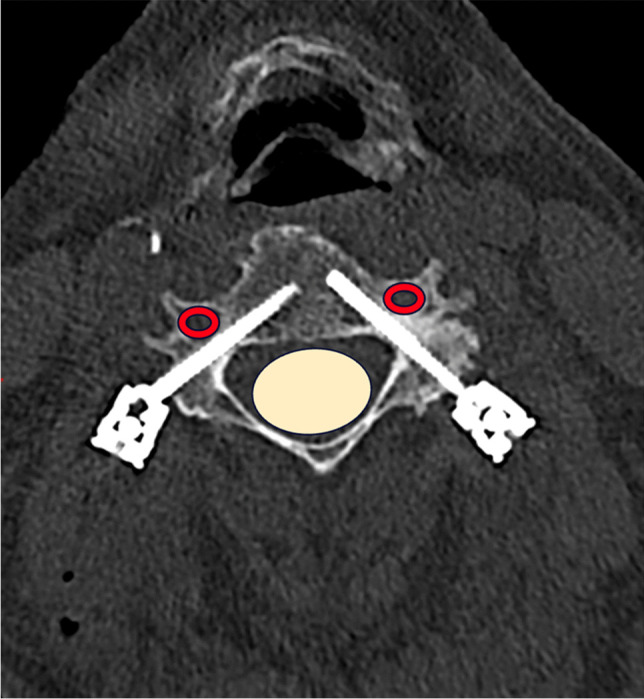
Postoperative CT image in the axial plane showing cervical pedicle screws in place, the vertebral arteries are marked in red, the spinal cord in yellow
